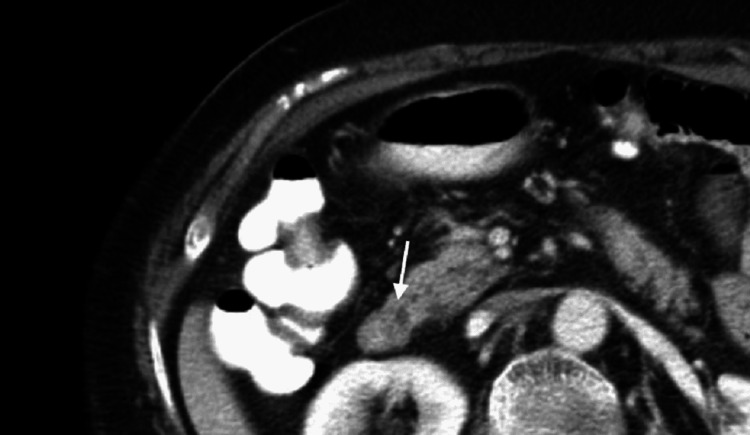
CT abdomen axial view in the venous phase shows a hypodense focal dilatation at the termination of the dorsal pancreatic duct, consistent with a Santorinicele (arrow).
